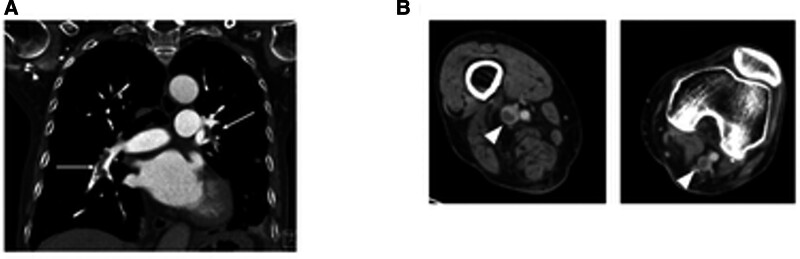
Contrast-enhanced computed tomography of venous thrombosis embolism. (A) Partial filling defect in the bilateral pulmonary arteries indicating pulmonary embolism (arrow). (B) Deep venous thrombosis in the bilateral popliteal veins (arrowhead).
